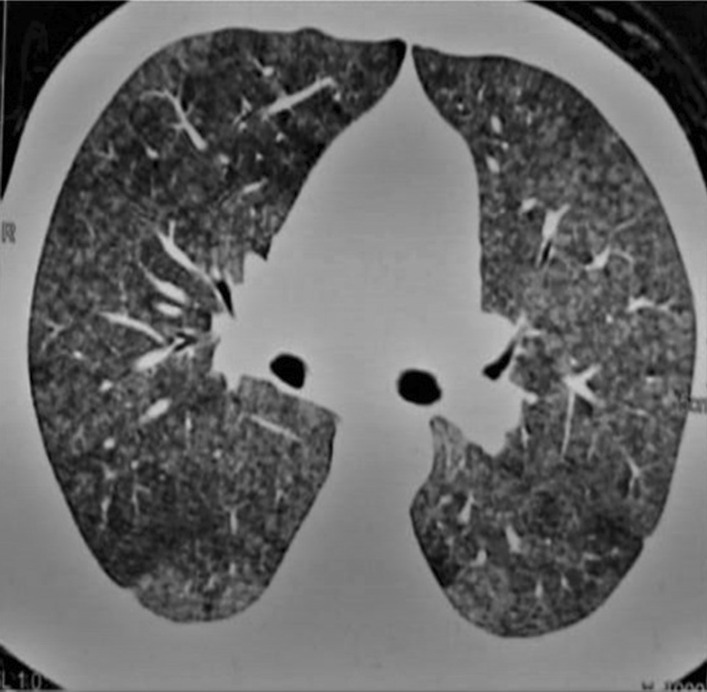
Female patient 36 years with nodular pattern in HRCT chest, low probability of PH in echocardiography and mild restriction spirometry
